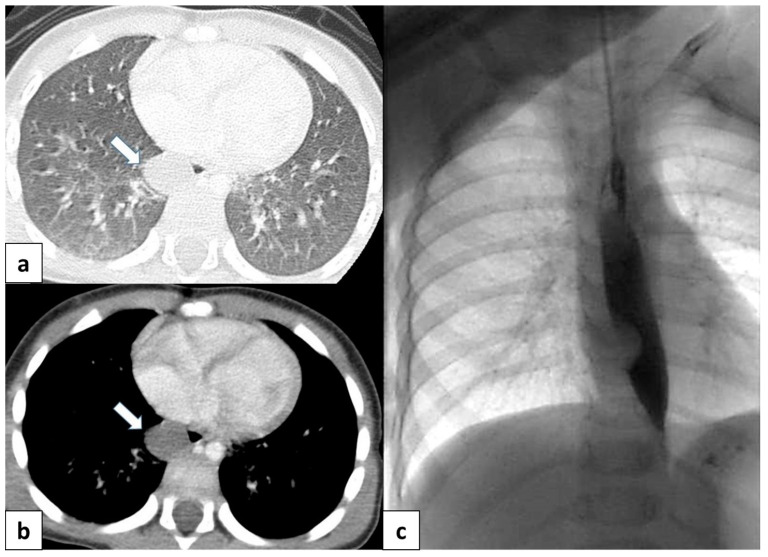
(a) CT scan in a 6 month old child shows a well-defined, rounded lesion with uniform fluid attenuation (arrow), compatible with a bronchogenic cyst; (b) after administration of intravenous contrast, bronchogenic cyst shows no enhancement or a minimally enhancing wall (arrow). (c) Dynamic esophageal fluoroscopy with oral contrast administration revealed a round, filling defect within the esophageal lumen, indicating that the cyst arises extrinsically from the bronchial tree.
